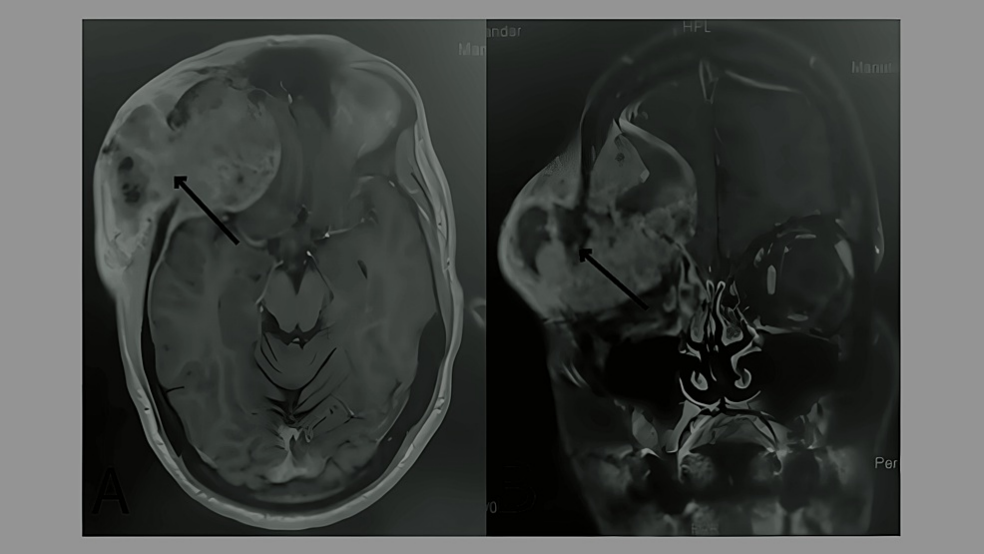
Axial and Coronal Sections of Brain MRI
